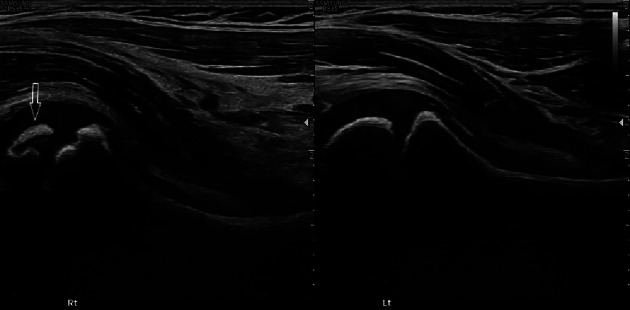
Ultrasound. Irregular delineation of the right proximal femur (arrow).
